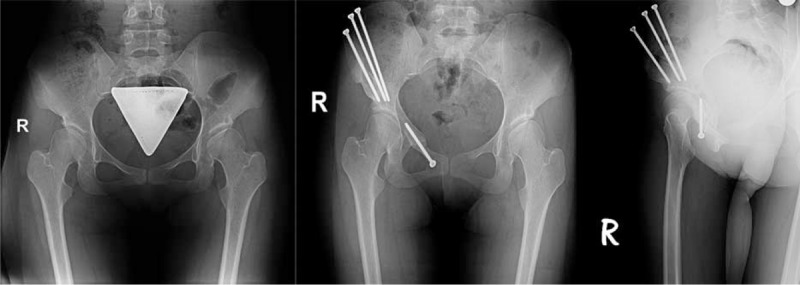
Patient 1: primary acetabular dysplasia; 21-year-old female. Preoperative and postoperative 3rd y AP and faux profile radiography.
